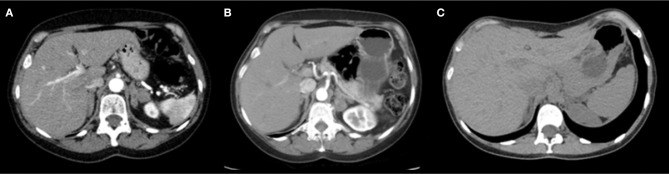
Abdominal CT scan with contrast showing. (A) Index-case: volumetric increase and diffuse nodular thickening of both adrenal glands, stable since 2011. (B) Index-case's sibling: bilateral enlargement of both adrenal glands with multiple hypoattenuating nodules, the largest measuring 2.5 cm (in the medial stem of the left adrenal), all with average attenuation of <10 HU in the non-contrast phase. Such nodules remain stable in relation to the tomographic study from 2015. (C) Index-case's niece: Both adrenal glands with preserved morphology and attenuation without identifiable focal lesions.
